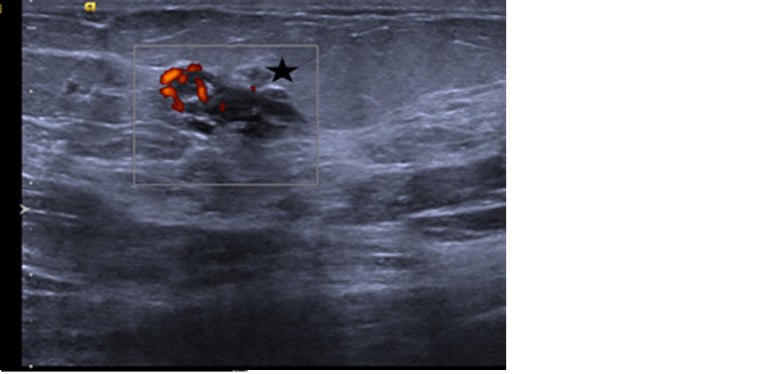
Right craniocaudal mammographic (A) and ultrasound images (B) of the case.
